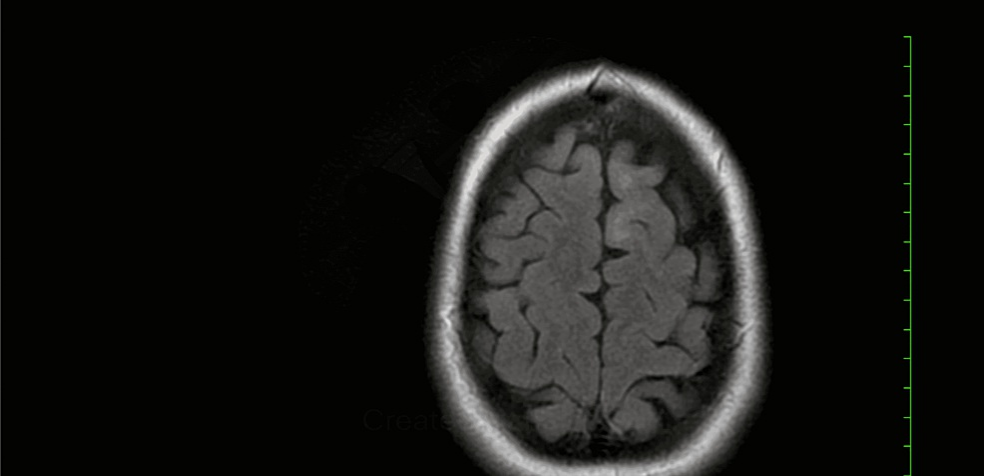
T2 MRI brain with and without contrast in six months shows resolution of demyelinating lesions with no new lesions.
